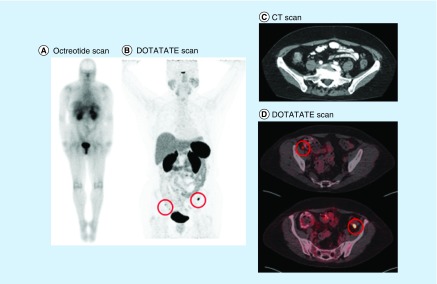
Representative imaging in a 65-year-old female who presented to our institution status post-small bowel resection with pathology showing neuroendocrine tumor, grade 2. She was considered free of disease at that time; however, she continued to have symptoms of diarrhea, flushing and fatigue. (A) Whole-body ^111^ln-octreoscan was negative. (B) Whole-body DOTATATE scan showing uptake in the right mesocolon and descending colon. (C) CT scan showing no positive findings (contrast and axial imaging). (D) DOTATATE scan showing uptake in right mesocolon and descending colon (axial imaging).
